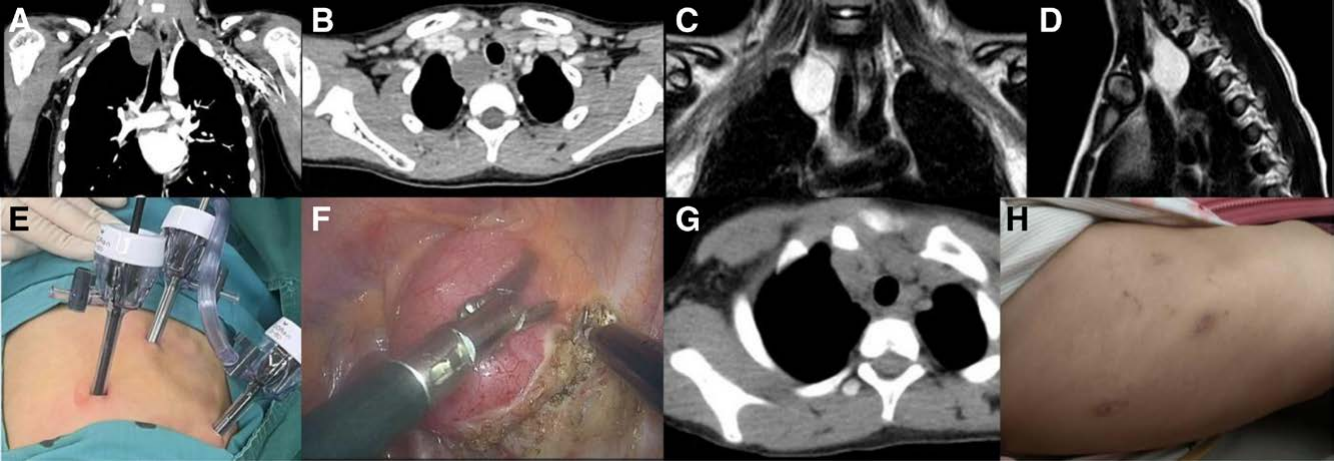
This is a 7.5-yr-old patient who was diagnosed with pneumonia through a chest CT scan. (A–D) The cysts can be seen to have caused compression of the surrounding tissues. Therefore, the parents insisted on surgery. (E) The surgical incision and the location of the puncture card can be seen in the image. (F) The cyst was removed during the operation. (G) Postoperative chest CT showed that the cyst had been completely removed. (H) The surgical wound healed well.
